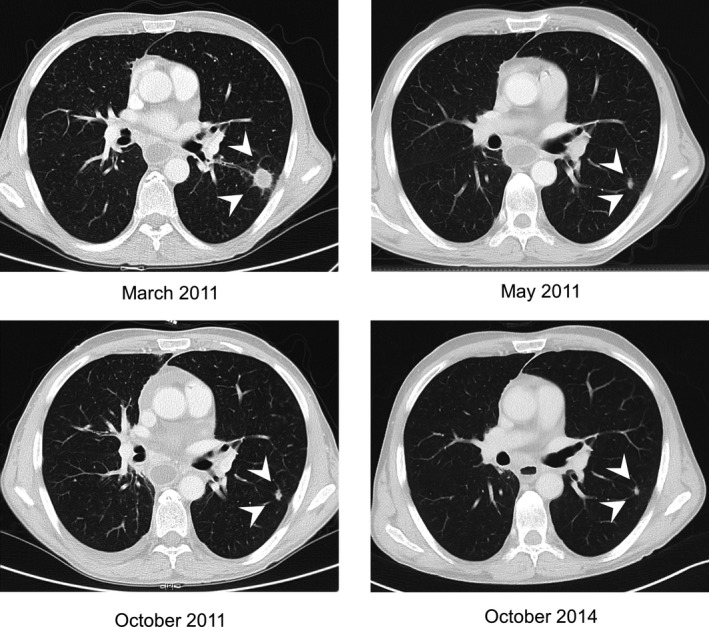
Computed tomography scans of a 49‐year‐old patient treated with topotecan after progression to platinum and etoposide. The patient was diagnosed with cervical and mediastinal lymph node metastases of neuroendocrine carcinoma in September 2010. At this timepoint, no suspicious pulmonary lesions could be detected. After 5 cycles of chemotherapy with cisplatin and etoposide as well as irradiation of the cervical and mediastinal lymph nodes the patient showed a new pulmonary metastasis in March 2011 (white arrowheads). From April until October 2011 the patient received a total of 8 cycles of topotecan resulting in a sustained PR without signs of tumor activity for more than 3 years.
